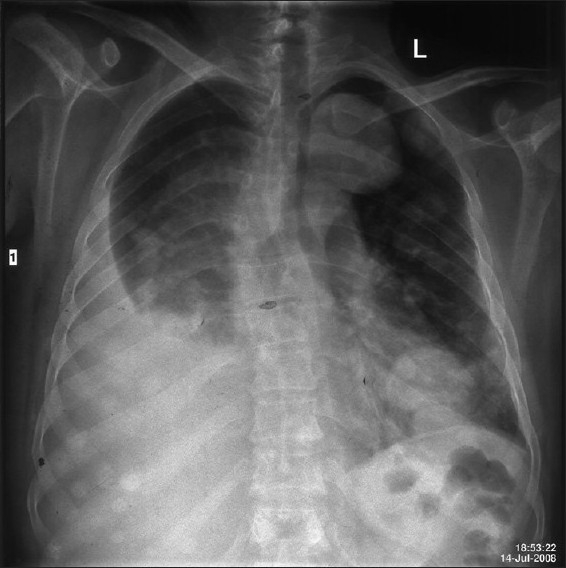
Chest X-ray showing nodular opacities in bilateral lung fields and pleural effusion on right side
